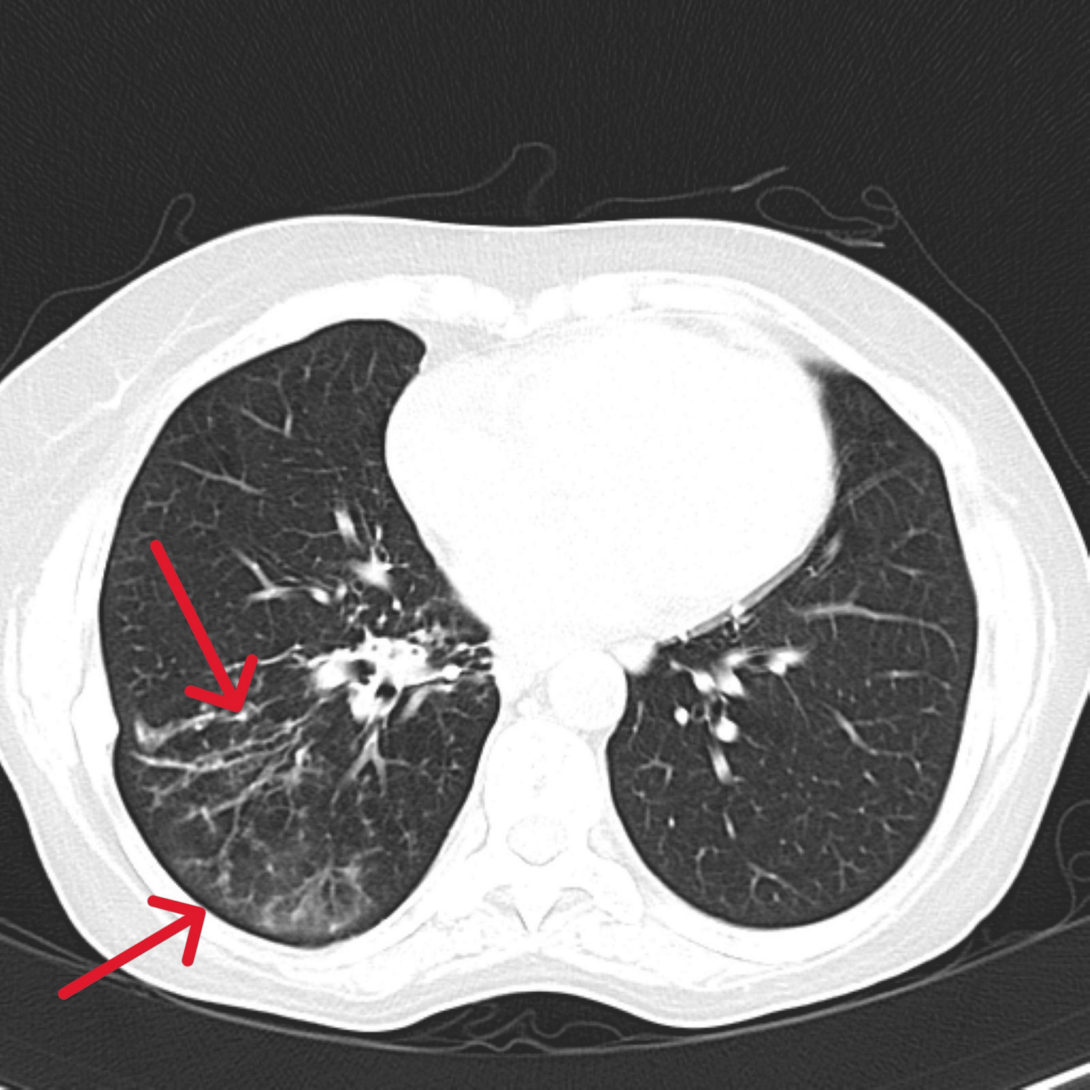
Axial section of a CT Chest. Case 3: Cylindrical bronchiectasis (red arrow) and nodularity of the right lower lobe, with ground glassing at the periphery.
